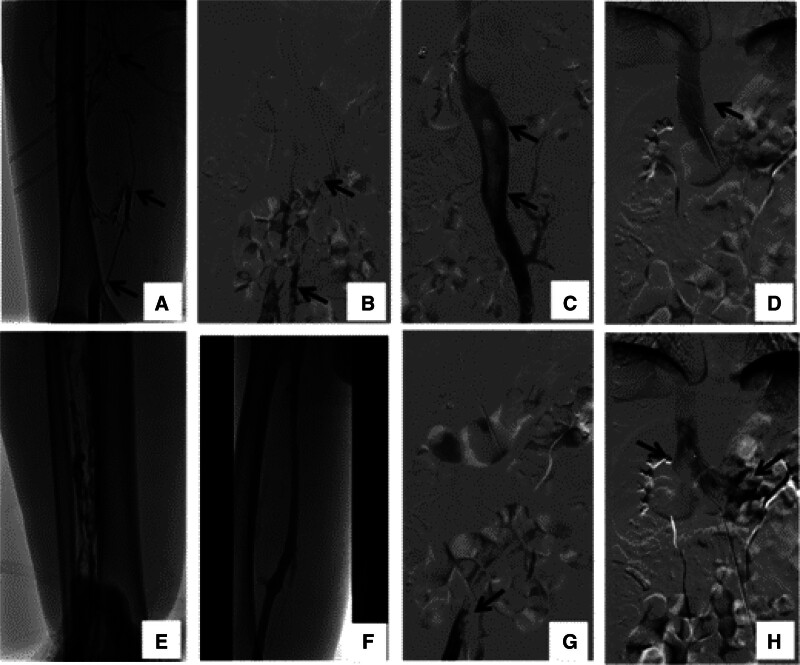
Contrast CT confirms the left-side IVC and no pulmonary artery embolism. (A) CTA showed iliac vein, iliac artery and left-sided IVC. (B) The IVC was left of the abdominal aorta. (C,F) Pulmonary CTA showed no pulmonary embolism. (D) The renal vein across the abdominal aorta. (E) The abdominal aorta on the left and the inferior vena cava on the right. CT = computed tomography, CTA = computed tomography angiography, IVC = left-sided inferior vena cava.
